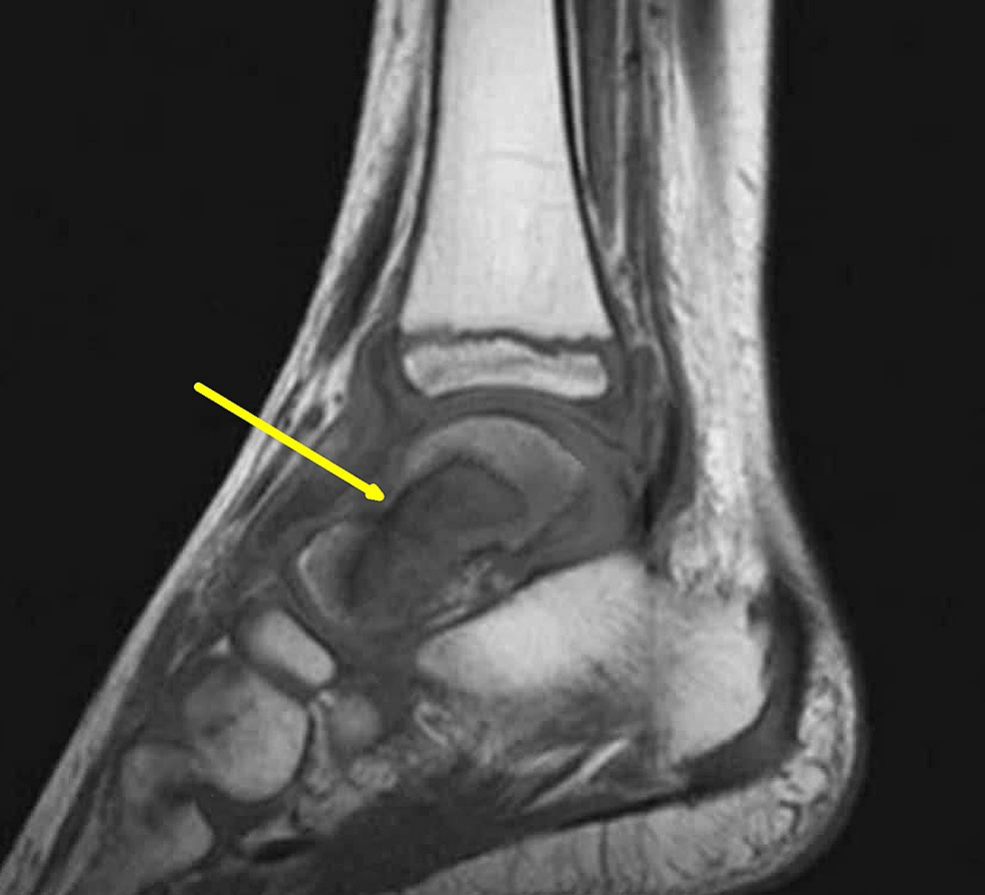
Magnetic resonance imaging of left ankle at presentation The T1 weighted image shows a hypointense lesion in the talus bone with surrounding marrow edema without any cortical breach (yellow arrow).
